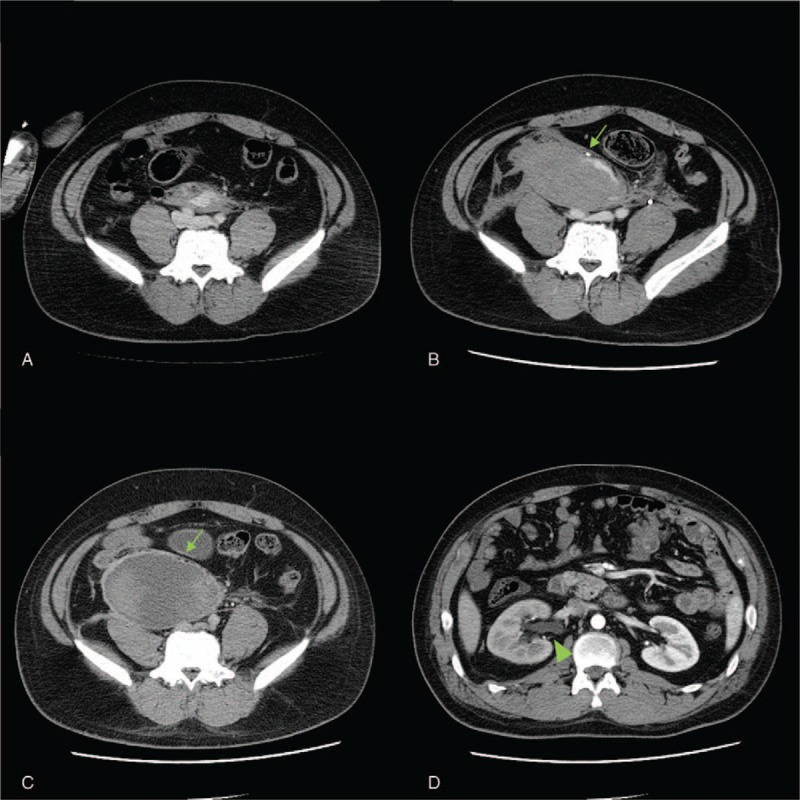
Time course of retroperitoneal hematoma on contrast-enhanced CT in the portal venous phase. Contrast extravasation was visible in front of the aortic bifurcation (A: on arrival). Contrast extravasation and hematoma expansion lifting the right ureter (arrow) was identified (B: 6 hours after arrival). The compressed right ureter (arrow) and dilatation of renal pelvis (triangle arrow) caused by hematoma growth was identified. (C, D: 24 days after injury). CT = computed tomography.
